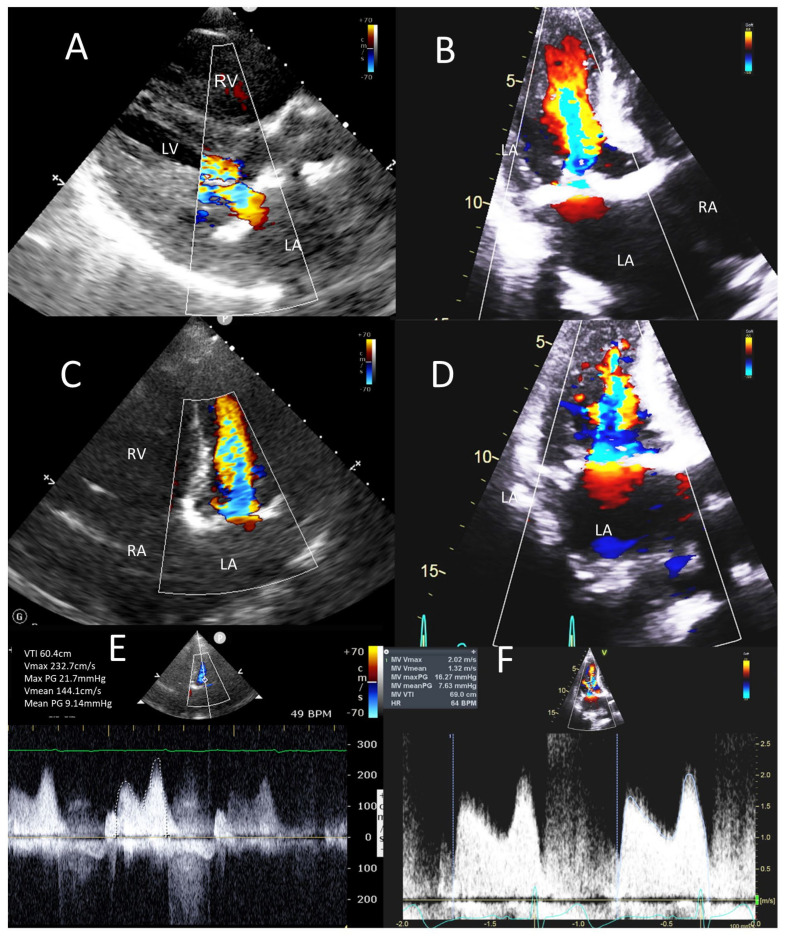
POCUS in stenotic mitral VHD. POCUS images (left-side panels) compared to the sTTE images (right-side panels) in the same patient as in Figure 6. Parasternal long axis view (A), apical 3 chamber view (B) and apical 4 chamber views (C,D) show turbulent mitral inflow on color Doppler. CW Doppler on mitral inflow in the 5 chamber view (E) and three chamber view (F) showed marked increase in mean mitral inflow gradient of 9 mmHg on POCUS (E) and 8 mmHg on sTTE (F). LA, left atrium; LV, left ventricle; RA, right atrium; RV, right ventricle.
